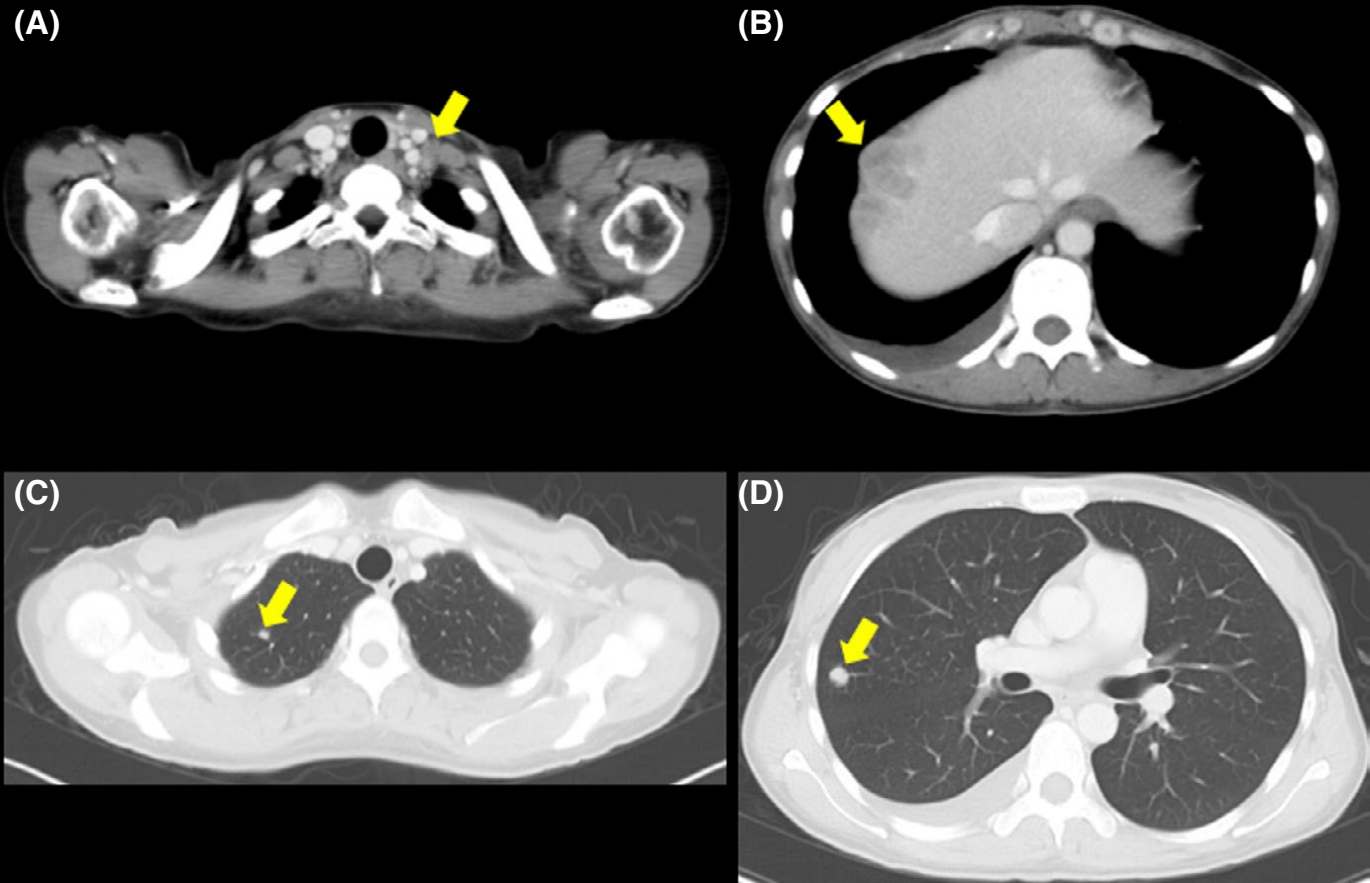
Computed tomography (CT) at 2 mo after completion of chemoradiotherapy. Metastatic tumors (arrows) in (A) the left supraclavicular lymph node, (B) the liver, and (C, D) the lung
